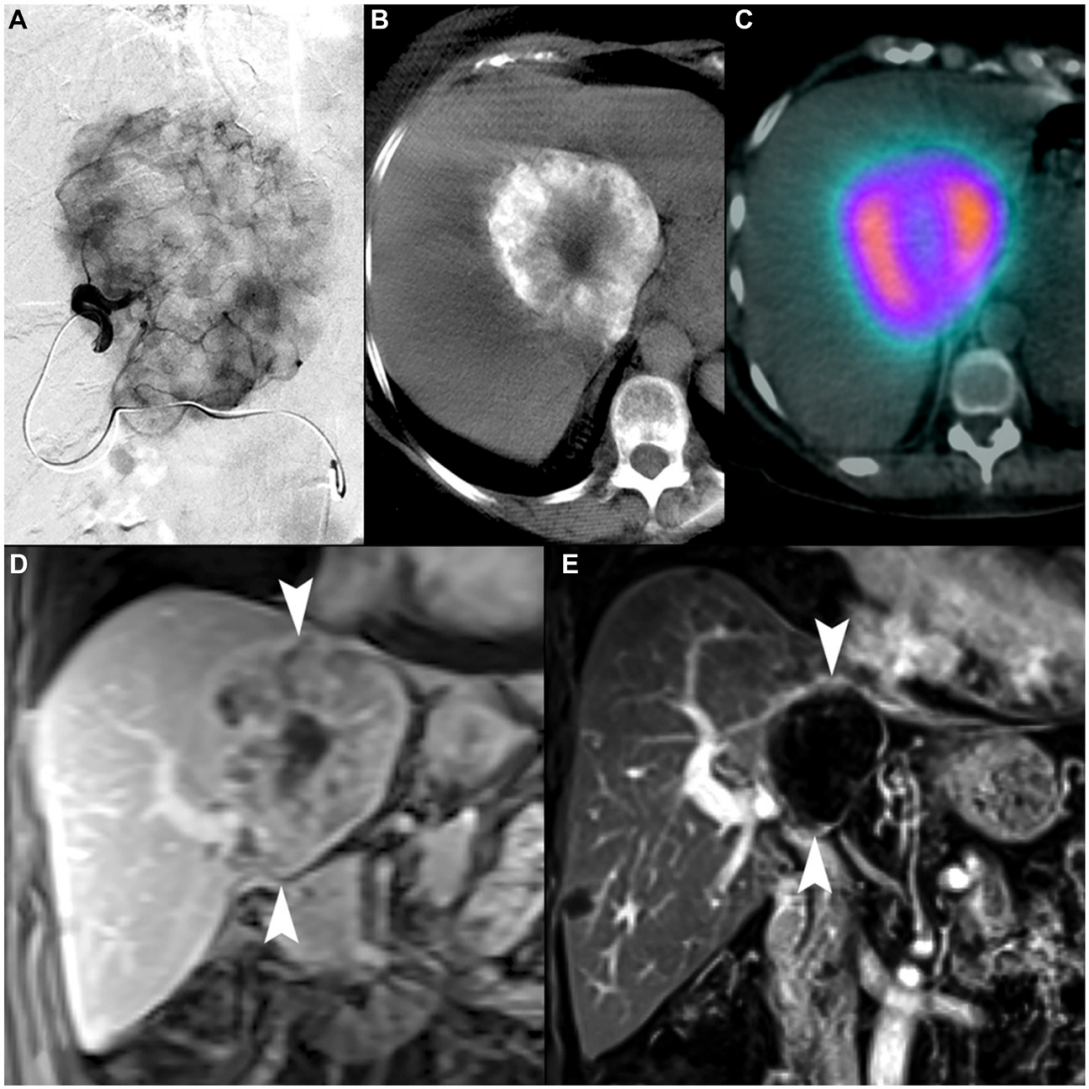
70-year-old female with intrahepatic cholangiocarcinoma. (A) Selective arteriography of a 9.2 cm iCCA demonstrating favorable macrovascular conduit for radioembolization. (B) Cone-beam computed tomography showed complete tumor coverage within two angiosomes (second not shown). (C) ^99m^Tc-MAA SPECT/CT demonstrated highly conformal tracer distribution with minimal exposure to non-tumoral liver. The MAA uptake within tumor (including the lower-activity necrotic center) demonstrates an example of favorable microvascular conduit due to overall relative uptake to normal liver parenchyma. (D) Coronal contrast-enhanced MRI image demonstrates the tumor at initial presentation (arrowheads). (E) Coronal contrast-enhanced MRI 3 years after single-session radioembolization with a dose of 476.8 Gy shows a persistent mRECIST complete response and contraction to 4.2 cm (arrowheads).
